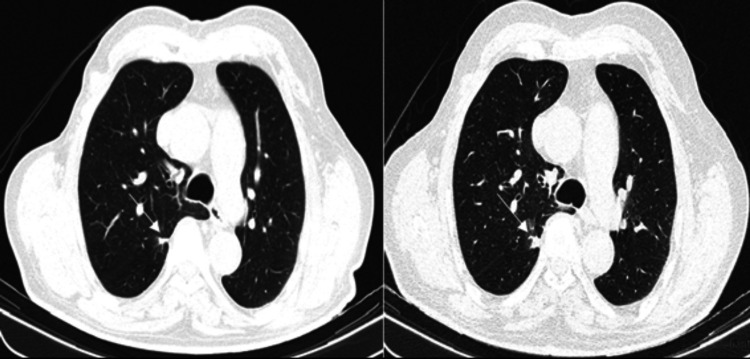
Nodule growth observed on CT scan of the thorax Left: Axial CT scan of the thorax (slice thickness: 3.00 mm, lung window) showing a solitary solid pulmonary nodule (white arrow) measuring 6 mm in the apical segment of the right lower lobe, with spiculated margins and pleural contact. Right: Corresponding axial CT scan one year later demonstrating interval growth of the same nodule (white arrow), now measuring 17 mm.
